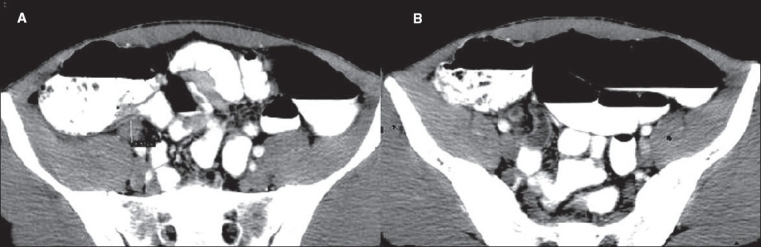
Appendicitis. Contrast-enhanced CT scan shows a thickened, fluid-filled tubular structure (arrow) in the right hemipelvis, contiguous with the cecal base
